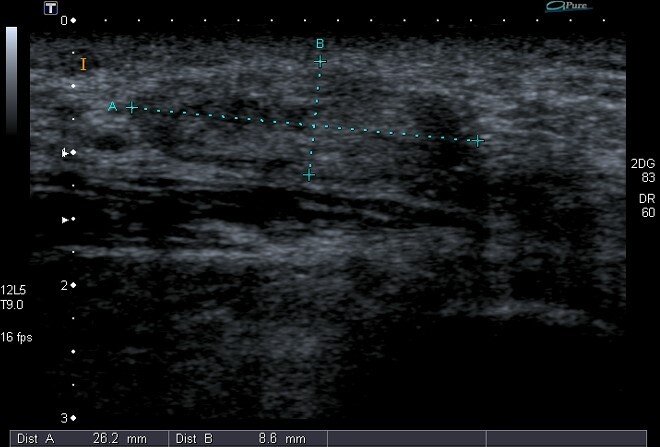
Longitudinal high-resolution greyscale ultrasound image of the perineal region just medial to the ischial tuberosity
